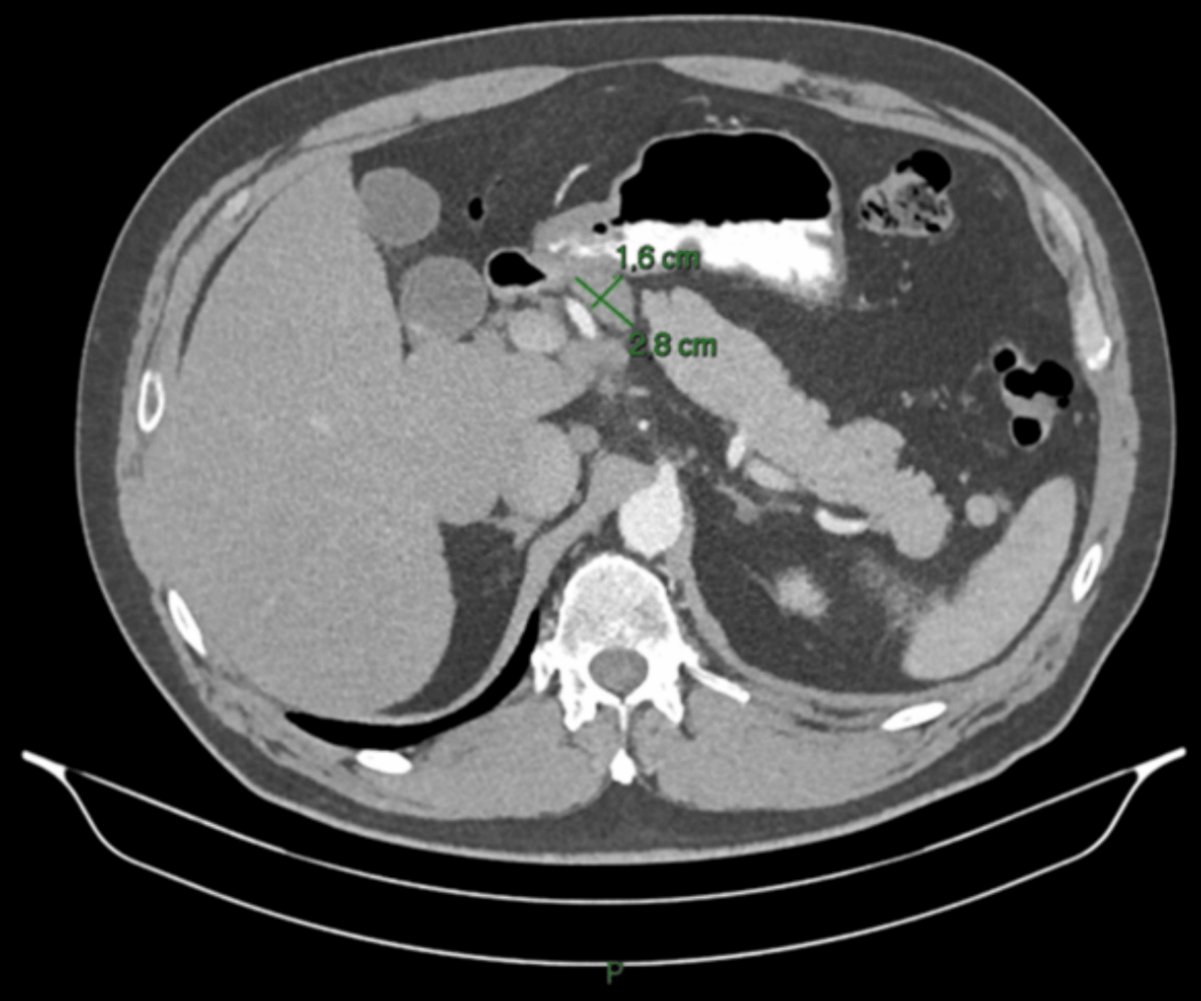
Follow-up CT abdomen (axial view), showing persistent lymph node near the gastric antrum (2.8 × 1.6 cm)
